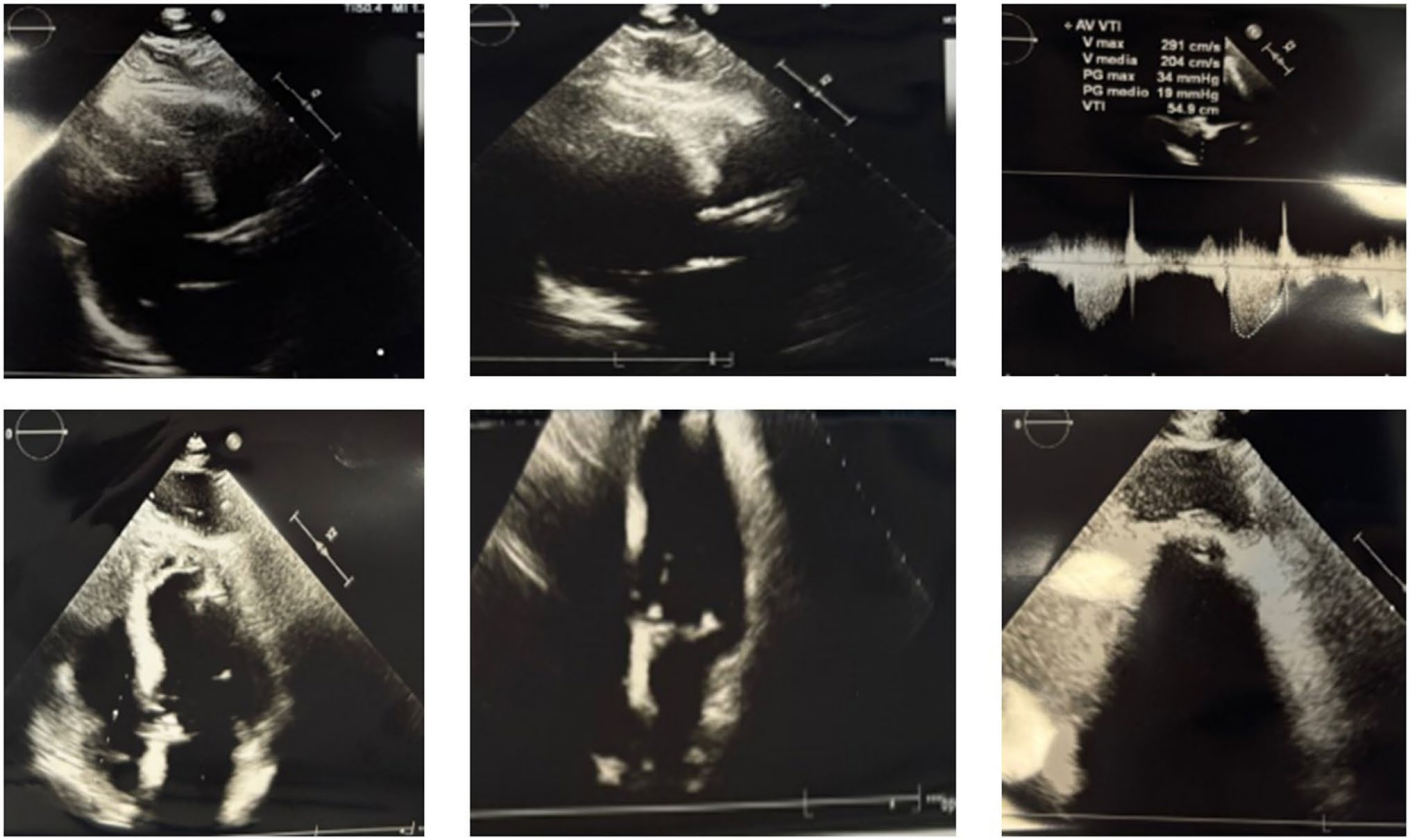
Echocardiogram of the patient who underwent a trans-apical antegrade TAVI procedure for aortic stenosis approximately 4 years ago. Images in different acoustic windows. The echocardiogram showed a slightly enlarged, hypertrophic left ventricle with normal contractility, together with the presence of a rounded image, with hyperechogenic margins and anechoic content, at the apical level of the left ventricle. The trans-prosthetic gradient was also measured at the TAVI level, which was found to be 19 mm Hg
